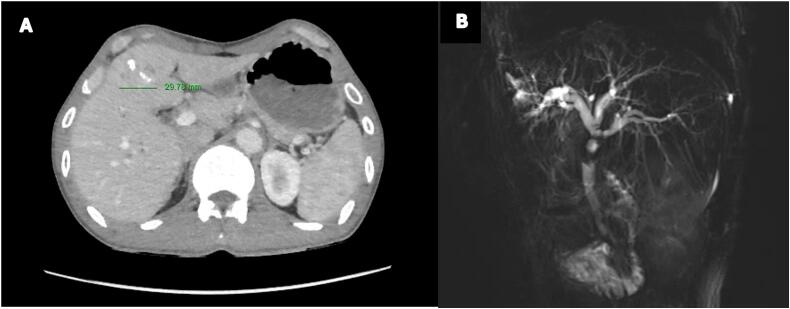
Pre-operative abdominal images: (A) Abdominal CT scan showed a tumor in the segment 4b with HCC characteristics; (B) Abdominal MRI showed the disruption at the biliary bifurcation combined with intrahepatic bile duct dilation.
